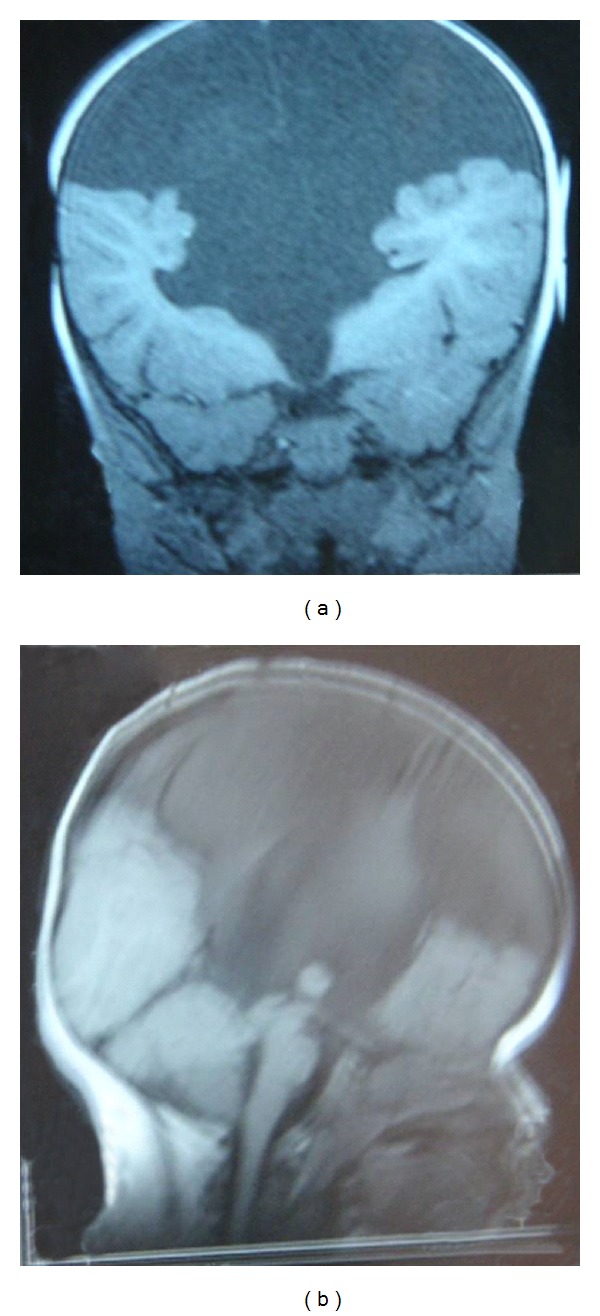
Magnetic resonance imaging of the brain revealing lobar holoprosencephaly.
